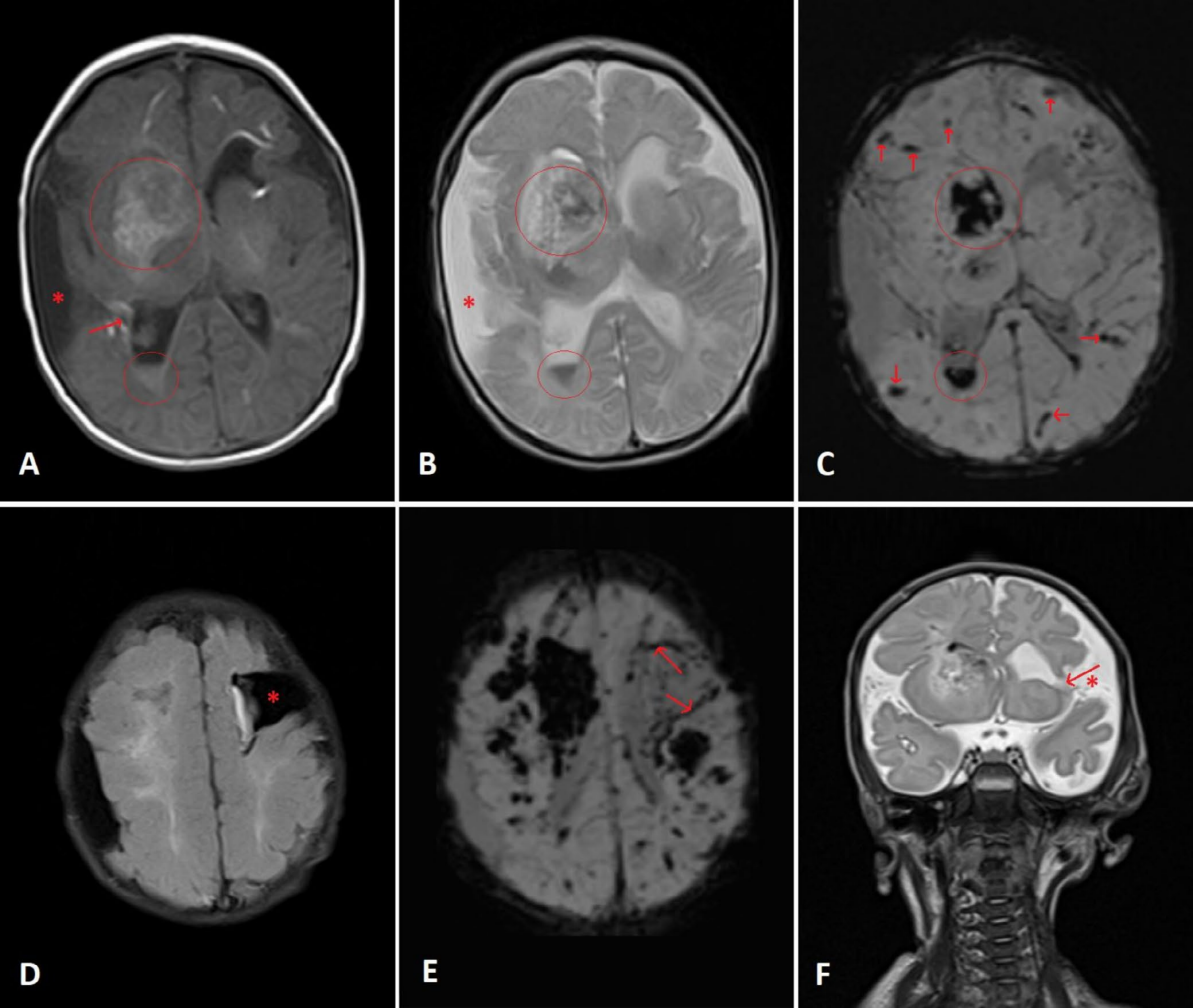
Multiplanar multisequence magnetic resonance imaging for patient 1's brain (A: axial T1WI, B: axial T2WI, C: axial susceptibility‐weighted images (SWI), D: axial fluid‐attenuated inversion recovery (FLAIR) image, E: axial susceptibility‐weighted images (SWI), F: coronal T2WI). The images show intraventricular hemorrhage mainly at the right lateral ventricle with intraparenchymal involvement of the right frontal periventricular area characterized by hyperintense T1 signal intensity (SI) (circles in A), hypointense T2 SI (circles in B) with blooming artifact at the SWI (circles in C), suggesting subacute hemorrhages. Other foci of blooming artifact are shown, representing microhemorrhages (arrows in C). An area of encephalomalacia is noted in the left fronto‐parietal region (asterisk in D & F), reaching the left lateral ventricle (arrow in F) as well as a surrounding rim of blooming artifact (arrows in E), suggesting superficial hemosiderosis. Another area of extra‐axial collection and encephalomalacia is seen in the right parietal region (asterisk in A & B) reaching the posterior horn of the right lateral ventricle (arrow in A). Findings mostly represent sequelae related to antenatal insults—likely hemorrhages.
